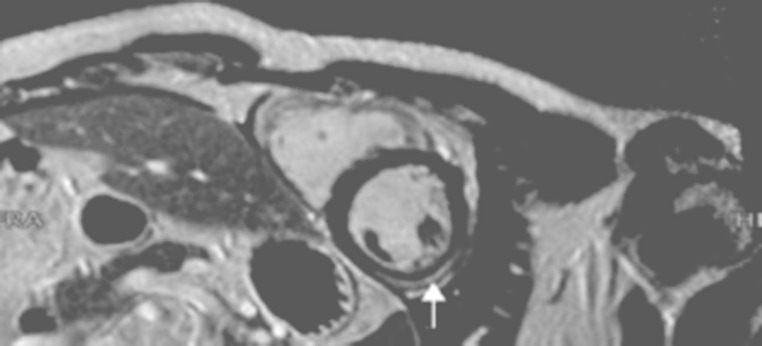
MR image of the patient. The white arrow (gadolinium contrast) points to subepicardial inflammation of the midwall, the clue to the diagnosis of myocarditis
